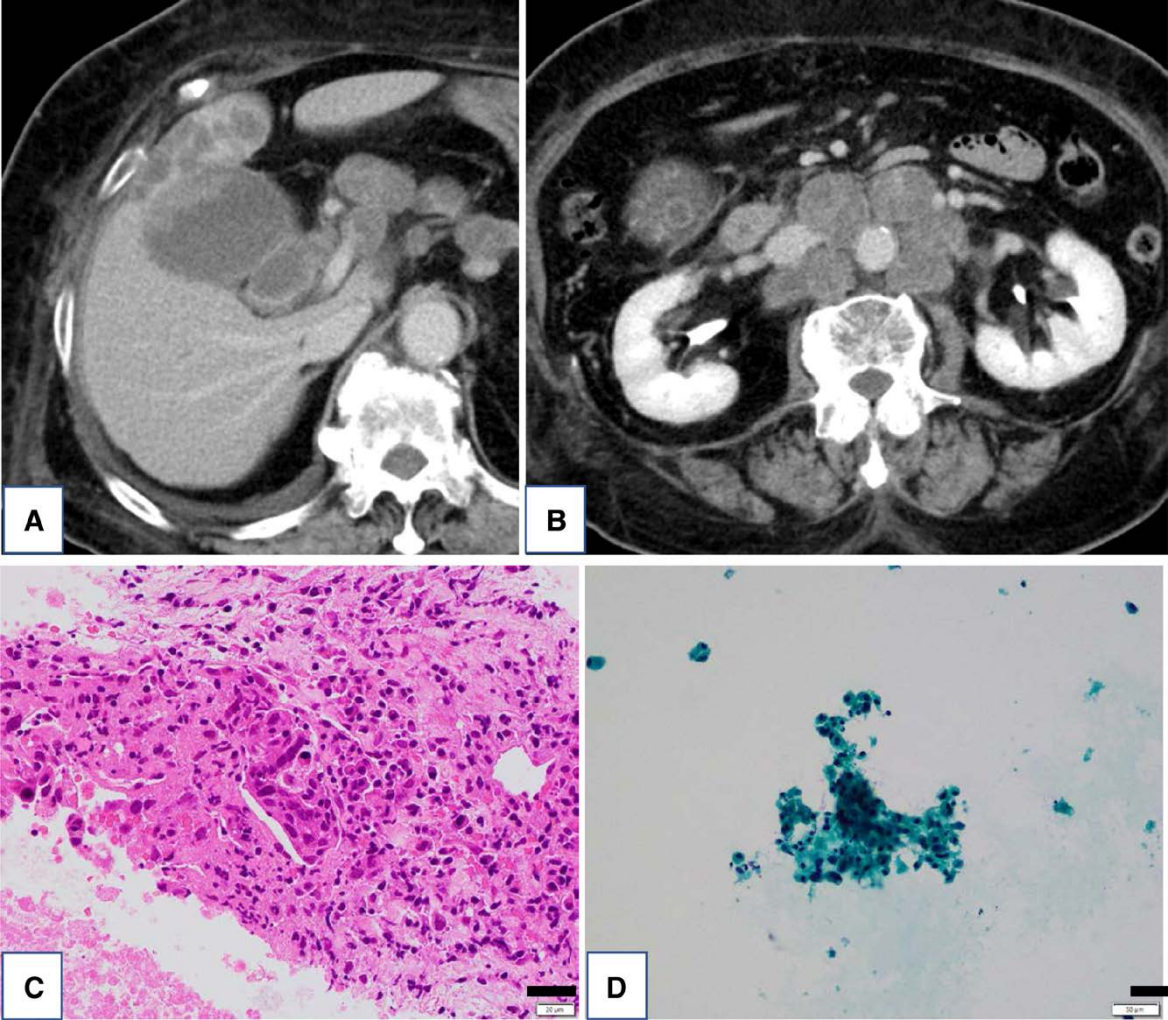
Abdominal CT images and cytopathological examination. There is a 4 × 1.5 cm mass in the body of the gallbladder with direct infiltration to segment 4 of the liver (A). Multiple lymph nodes around the abdominal aorta are swollen (B). Poorly differentiated adenocarcinoma is evident on cytological examination of species obtained from liver biopsy (C). Poorly differentiated adenocarcinoma is also observed on cytological examination of species obtained from a fine-needle aspiration using endoscopic ultrasonography (D). Scale bars: 50 µm (C), 20 µm (D).
